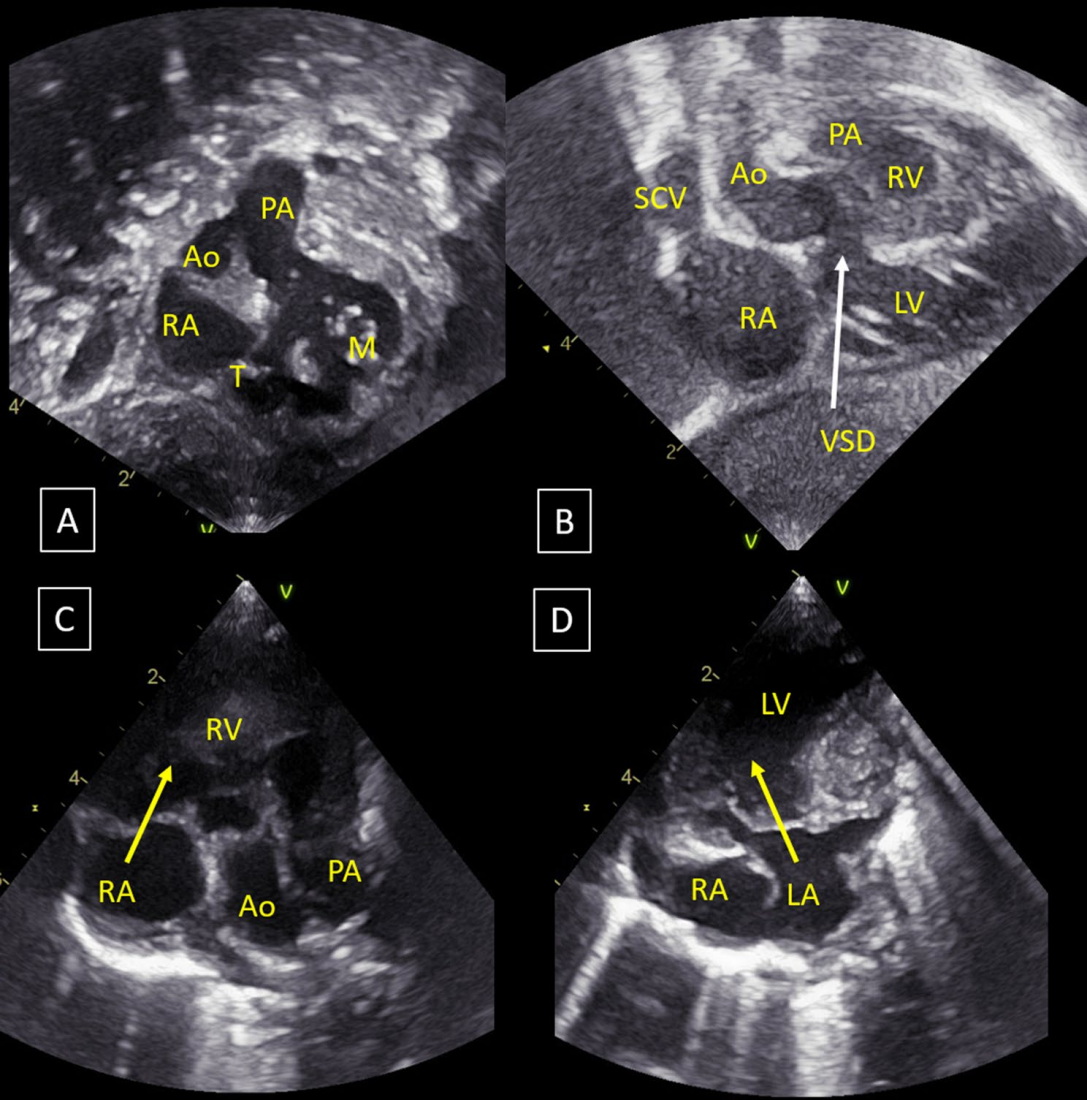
Ultrasound of criss-cross heart with supero-inferior ventricles, double outlet right ventricle (S,D,D), non-committed inlet VSD, hypoplastic right ventricle. (A): subcostal view, derived from coronal view. Mitral and tricuspid valves are in orthogonal planes; (B): sub costal view, coronal view: horizontal interventricular septum (supero-inferior ventricles); (C): 4 chamber view, right atrium-right ventricle connection (yellow arrow); (D): 4 chamber view, left atrium-left ventricle connection (yellow arrow); the two yellow arrows are in orthogonal planes. Ao, aorta; LA, left atrium; LV, left ventricle.; M, mitral valve; RA, right atrium; RV, right ventricle; T, tricuspid valve;; PA, pulmonary artery; VSD, ventricular septal defect
